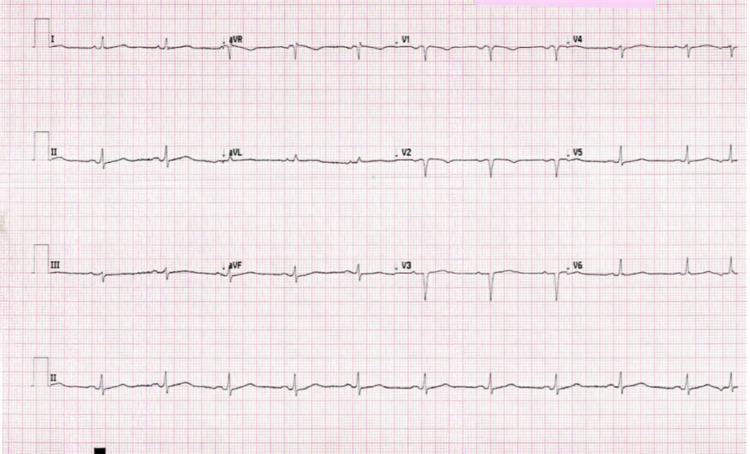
Echocardiogram showing sinus rhythm, poor R wave progression with Q waves, a premature atrial contraction, biphasic P waves, and low voltage.
